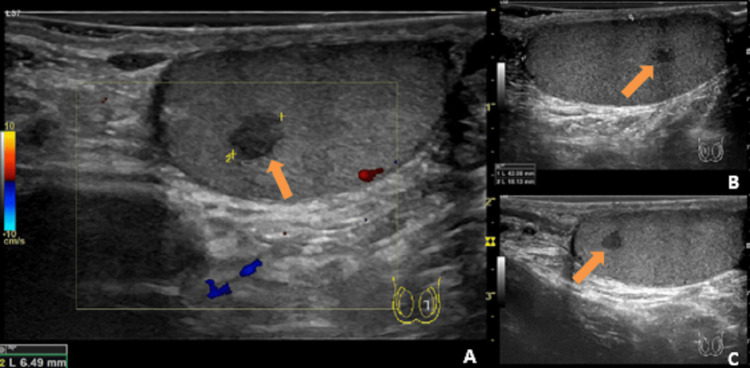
Images of the scrotal ultrasound showing three nodular lesions (orange arrows) A: One nodular lesion on the left testicle, B & C: Two nodular lesions on the right testicle
